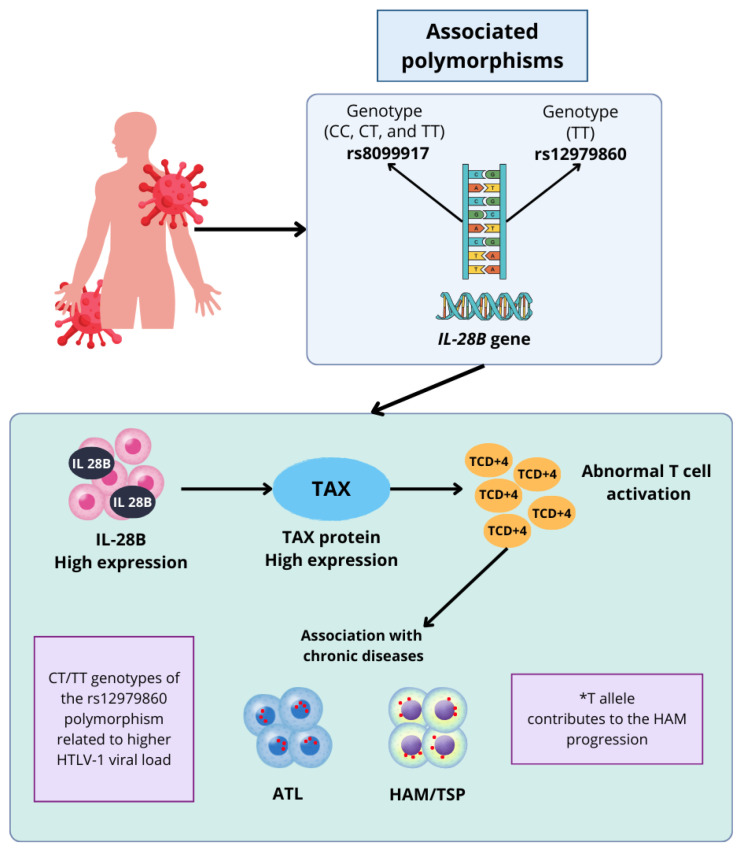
The role of *IL-28B* gene polymorphisms in HTLV-1 infection and associated diseases. *T refers to the T allele of the rs12979860 polymorphism of the *IL-28B* gene, specifically emphasizing this allele and not the complete genotype, such as CT or TT. Highlighting its possible relationship with the pathogenesis of the HTLV-1 infection and its influence on the development of associated diseases, the use of the asterisk indicates precisely that the individual effect of the T allele on the progression of the infection is discussed.
